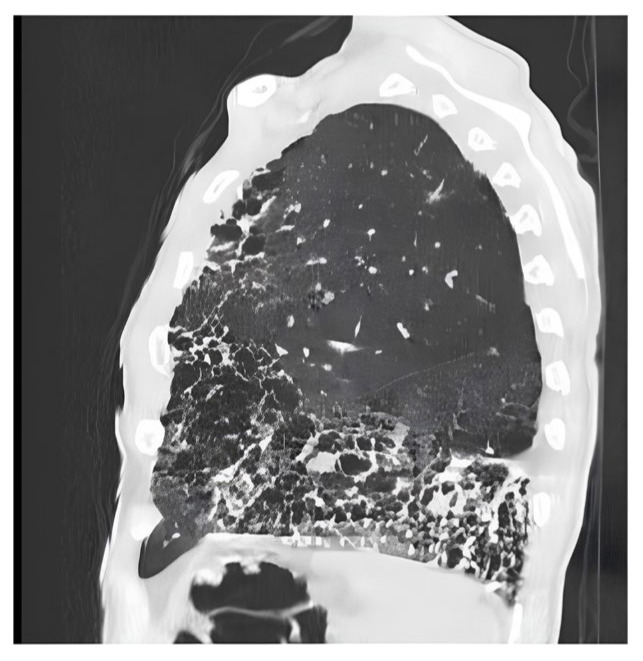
Sagittal section of a chest CT showing a scan appearance of definite Usual Interstitial Pneumonia (UIP). The abnormalities show a clear subpleural and basal predominance, with the most severe changes concentrated in the lower lobes of the lung. The hallmark of this pattern, extensive honeycombing, is clearly visible as clustered, thick-walled cystic airspaces arranged in multiple layers. In addition to honeycombing, there are prominent reticular opacities and evidence of traction bronchiectasis, representing the irreversible bronchial and bronchiolar dilatation caused by surrounding fibrous tissue. The combination of these findings, particularly the presence of honeycombing in a subpleural and basal distribution, is highly specific for the UIP pattern.
